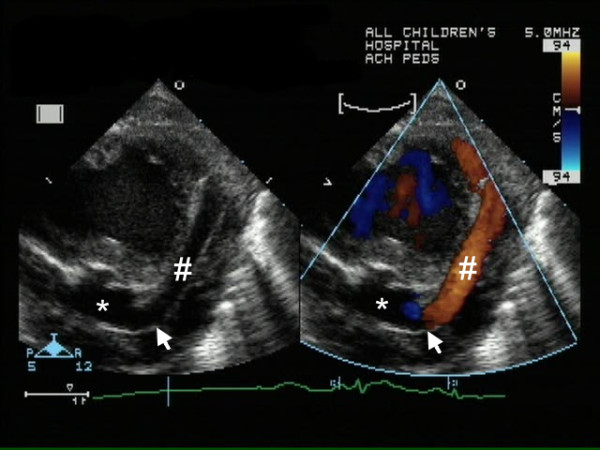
Two dimensional (left) and color Doppler (right) on day one (atypical short axis view of LV). The large coronary artery fistula drains (arrow) into the left ventricular apex (*). The LAD (#) was dilated up to 4.4 mm in diameter along its course.
